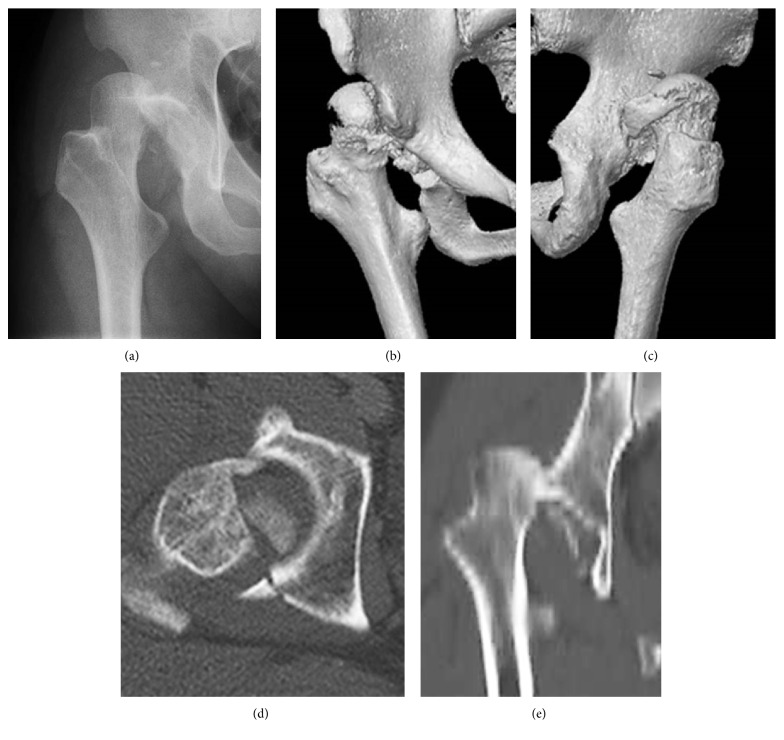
Case 1. A 45-year-old man with Pipkin type-IV fracture of the right hip with posterior dislocation of the femoral head. (a) Anteroposterior X-ray image of the right hip at the injury. (b) Anteroposterior three-dimensional computed tomography showing dislocation and fracture of the femoral head. (c) Posteroanterior three-dimensional computed tomography showing displacement of the posterosuperior wall and rim. (d) Axial computed tomography showing fracture of the femoral head and posterior wall of the acetabulum. (e) Coronal computed tomography showing dislocation and fracture of femoral head.
